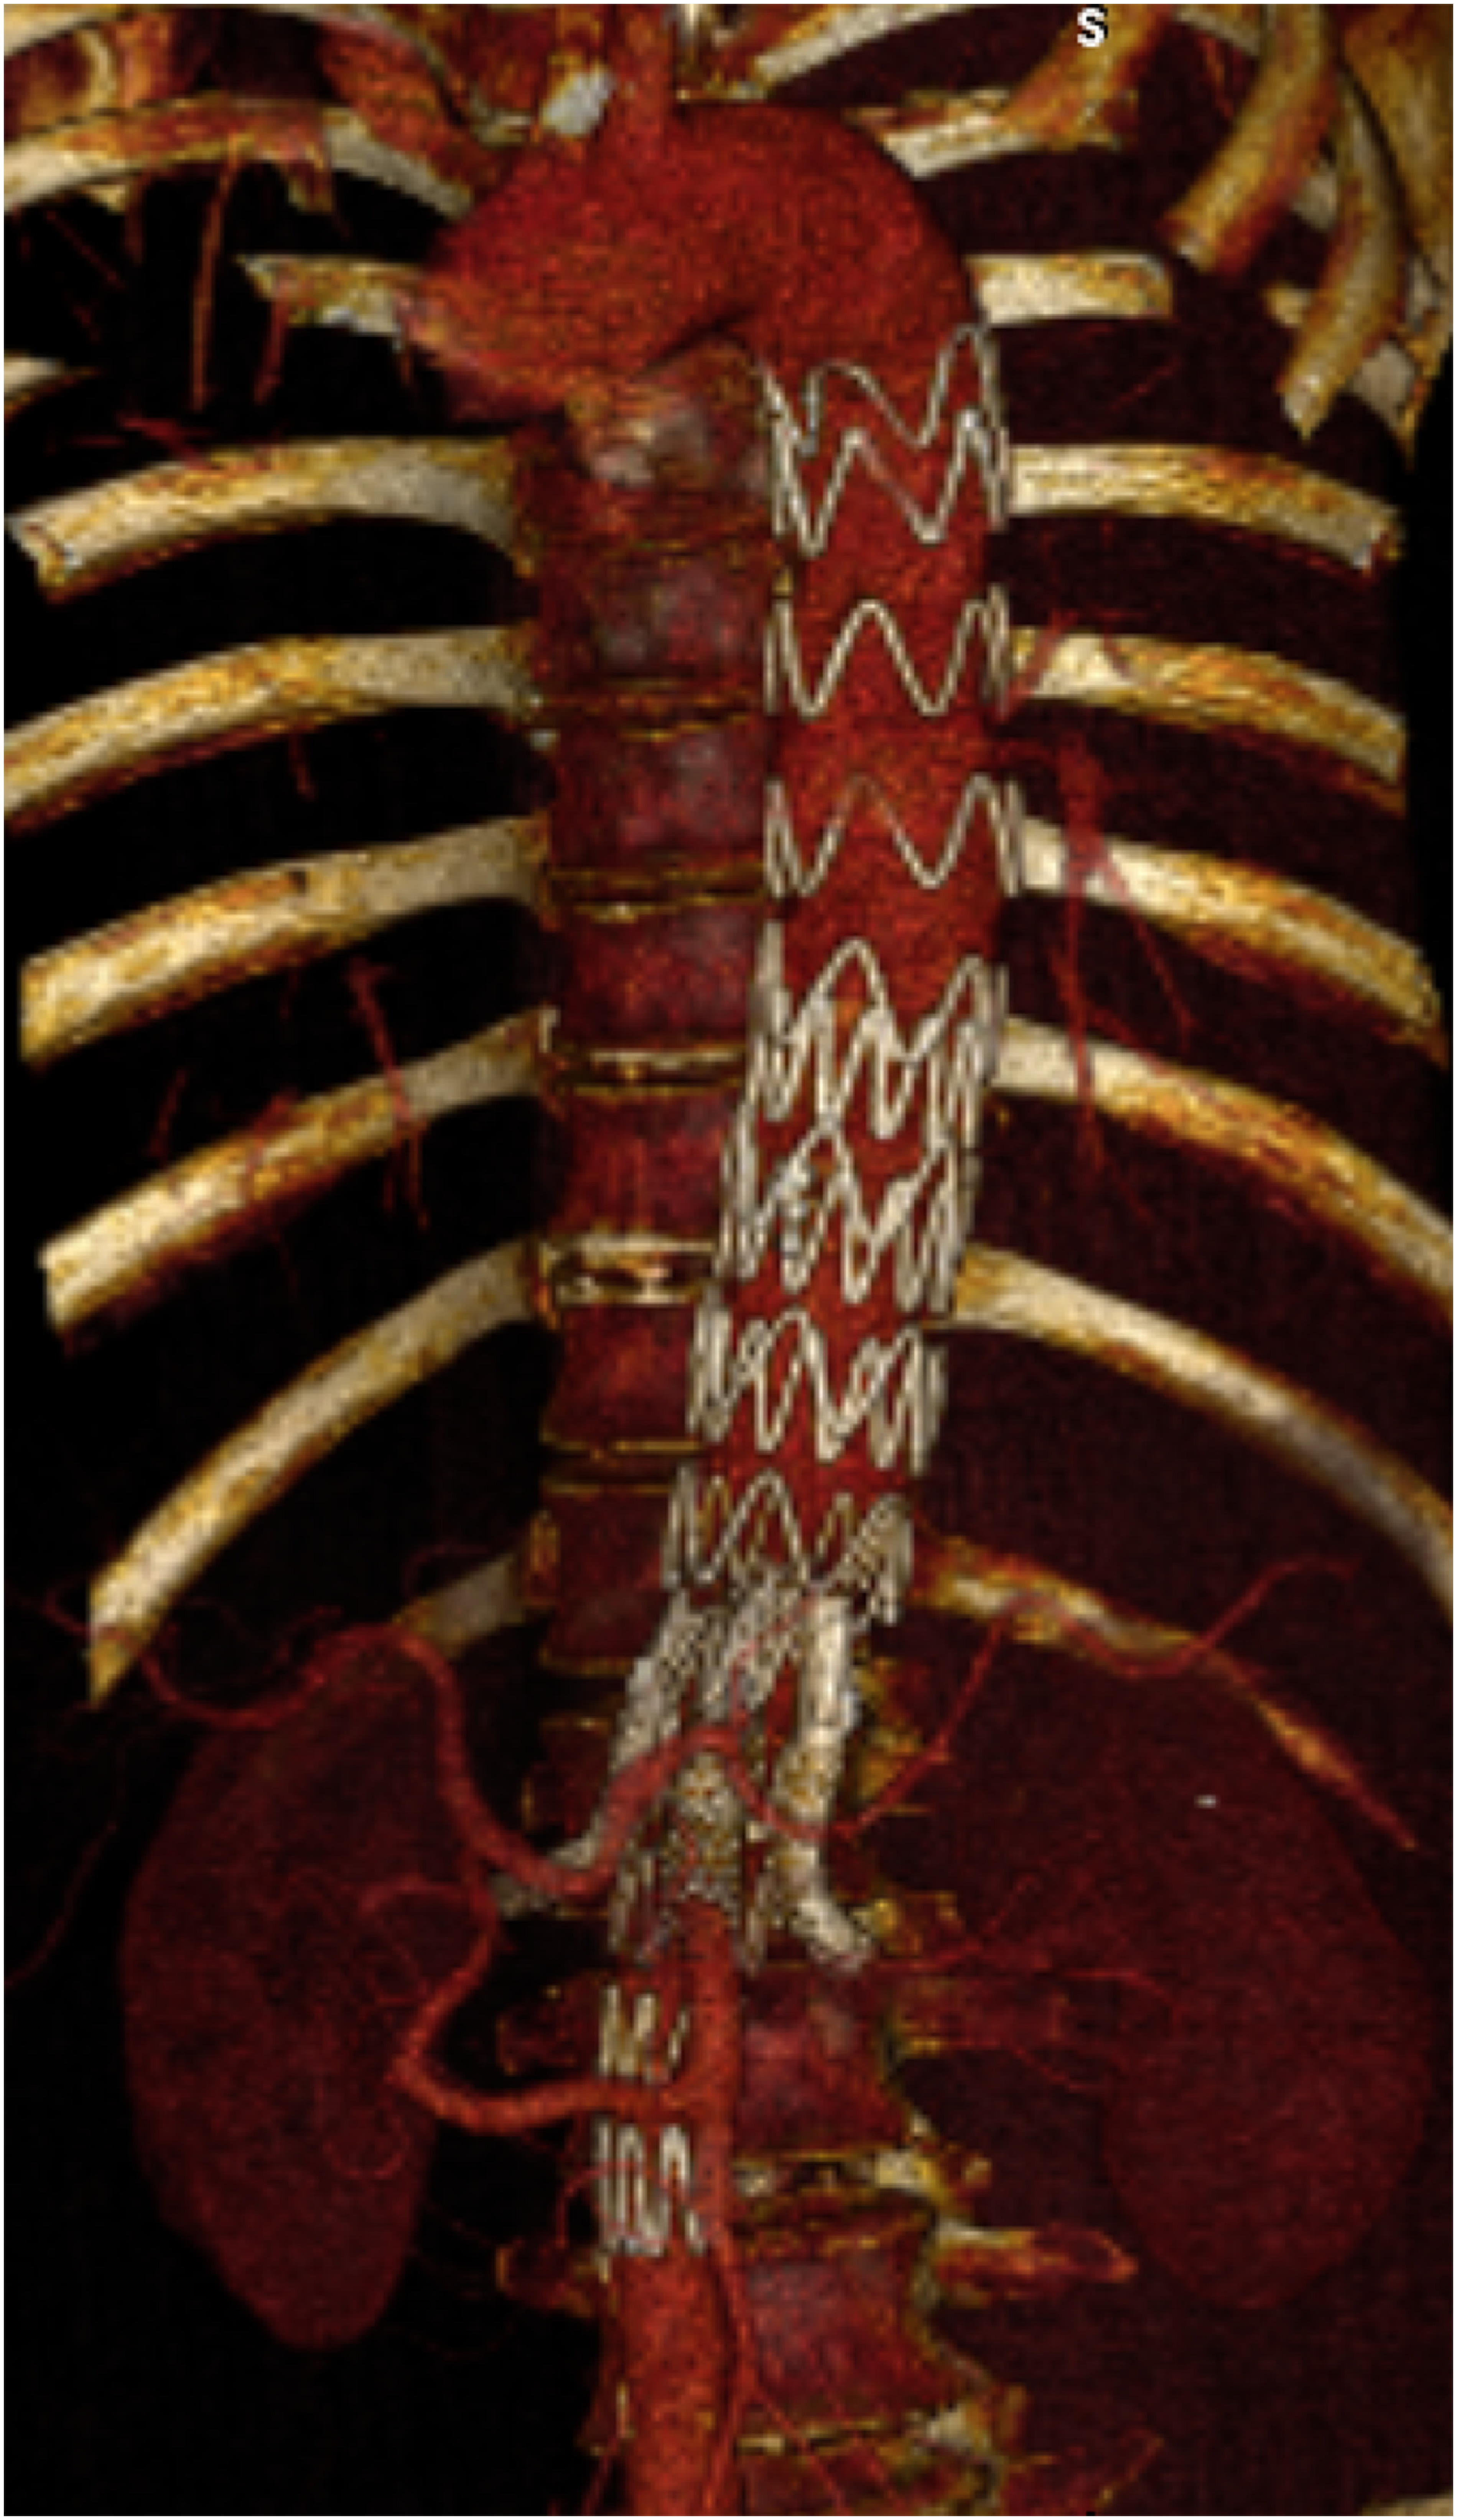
Fig. 3 Computed tomography angiogram 3 months post procedure.
